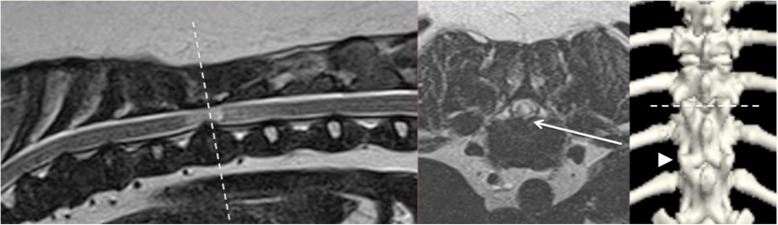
Magnetic resonance imaging of the thoracolumbar spine of case no. 6, displaying extra-dural spinal cord compression associated with T11–12 disc protrusion and concurrent bilateral T11 caudal articular process aplasia. From left to right; T2-weighted mid sagittal of thoracolumbar spine, T2-weighted transverse at the affected level T11–12 (transverse level marked with a dashed line), three-dimensional reconstruction of thoracolumbar junction on CT (T11–12 marked with dashed line; the normal articular processes of T12 are highlighted with an arrow head). There is a marked focal increase in T2-weighted signal within the spinal cord despite only mild ventral extra-dural spinal cord compression (arrowed on transverse image)
